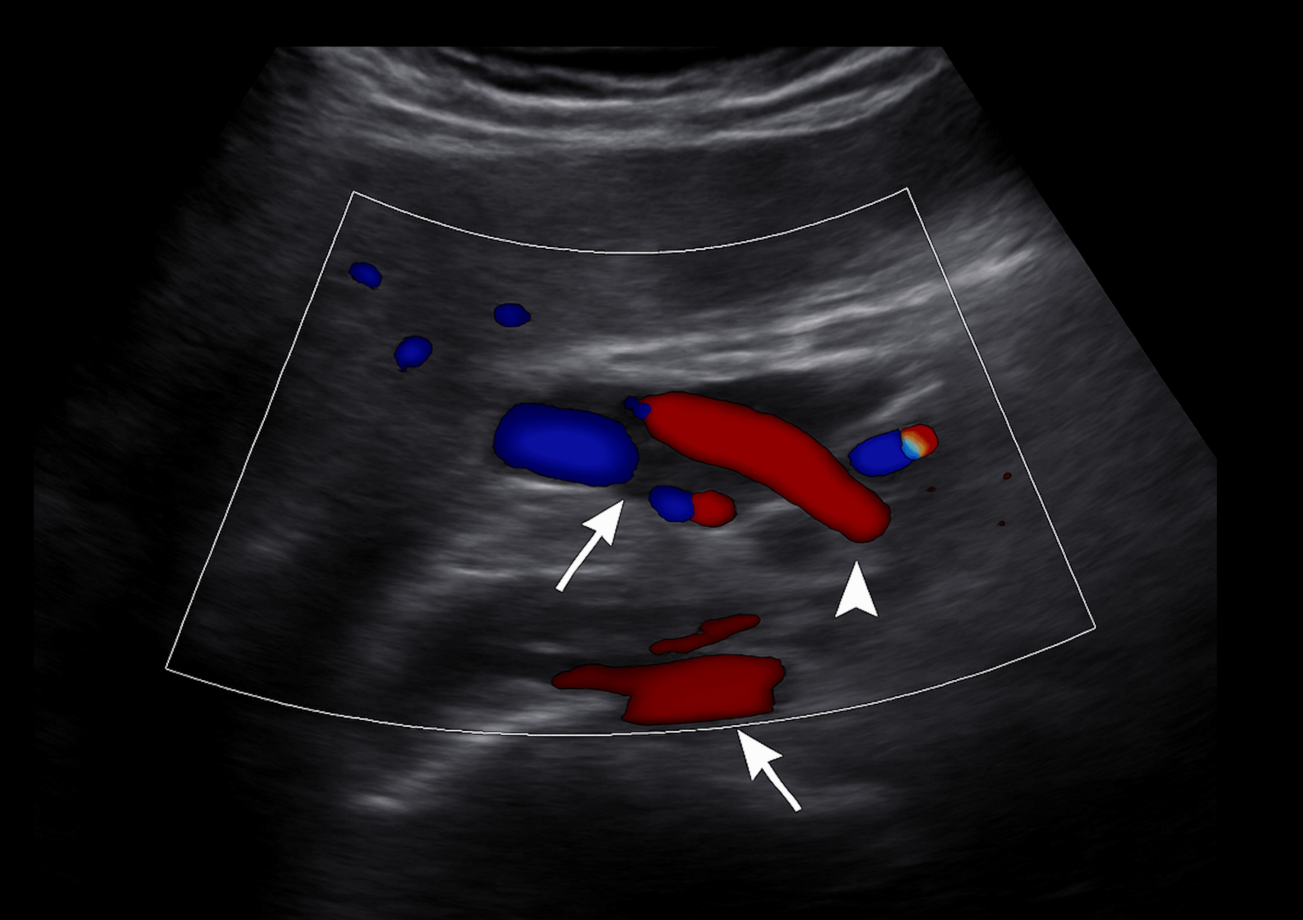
Doppler ultrasound image showing the intrapancreatic portion of the common bile duct (arrow) and the pancreas (arrowhead)
